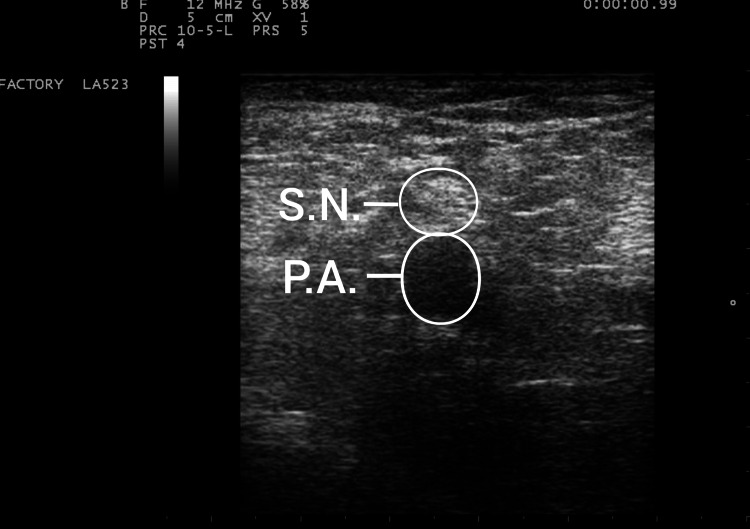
Sonographic picture of the popliteal sciatic nerve. SN, sciatic nerve; PA, popliteal artery
